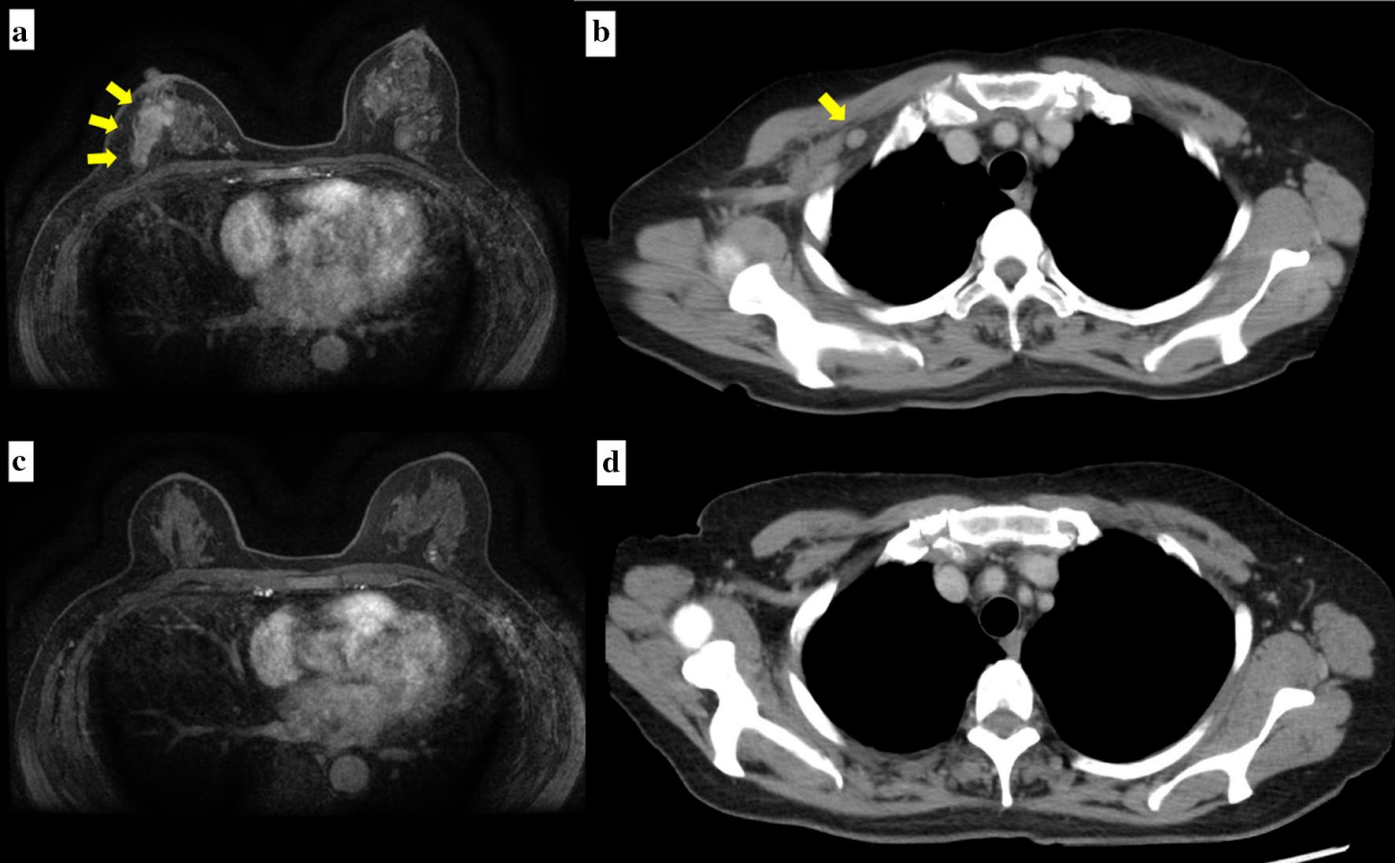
a MRI (T2-weighted image) showed a high-signal mass in the right breast before chemotherapy. b CT showed a swollen axillar lymph node before chemotherapy. c MRI (T2-weighted image) showed that the mass had disappeared after chemotherapy. d CT showed that the lymph node had disappeared after chemotherapy
